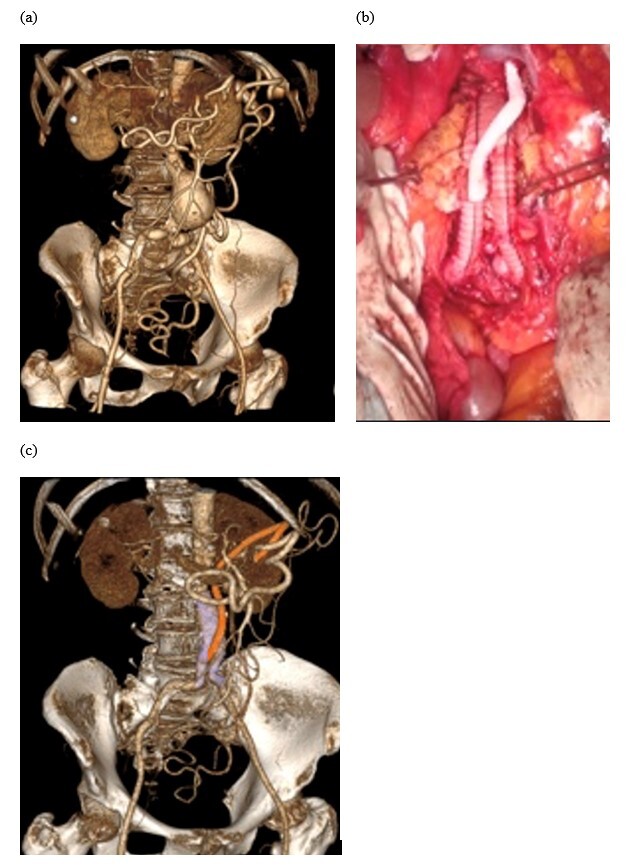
Intraoperative and imaging findings for patient #4. (a) Preoperative computed tomography angiography (CTA) with all 3 trunks occluded. Riolan’s arcade is filled by the left internal iliac artery; (b) Polyester aortobiiliac graft for correction of an abdominal aortic aneurysm with a reinforced PTFE graft from the right graft limb to the median colic artery at Riolan’s arcade; (c) Postoperative CTA showing the patent graft (highlighted in orange) and the polyester aortobiiliac graft (highlighted in purple).
